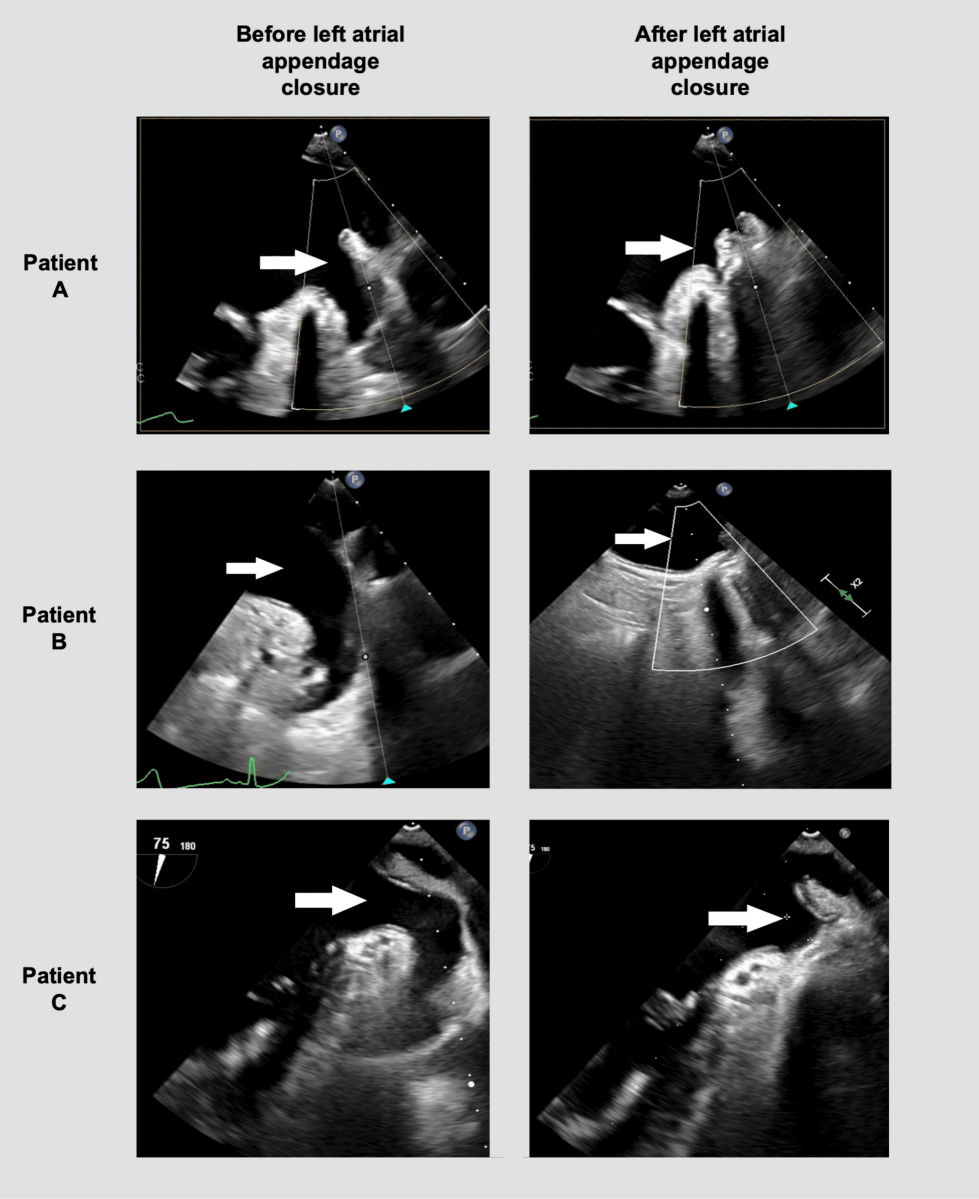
Intraoperative transesophageal echocardiography before and after the left atrial appendage closure of all patients. White arrows mark the left atrial appendage.
